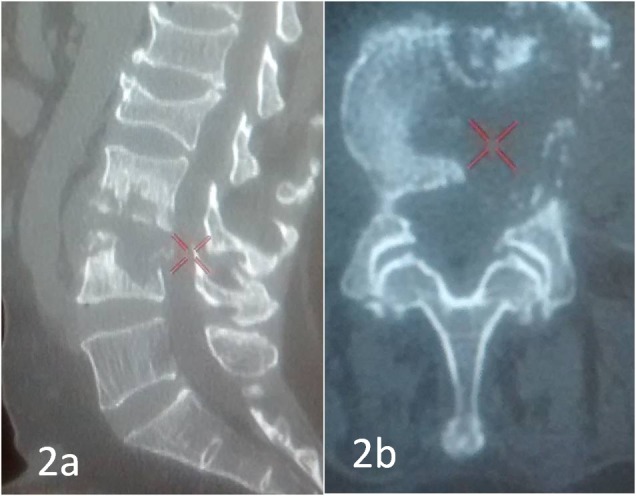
Sagittal and axial CT scans showing lytic destruction of the L3-4 vertebral bodies Sagittal and axial computed tomography (CT) scans showing lytic destruction of the L3-4 vertebral bodies (red X)
